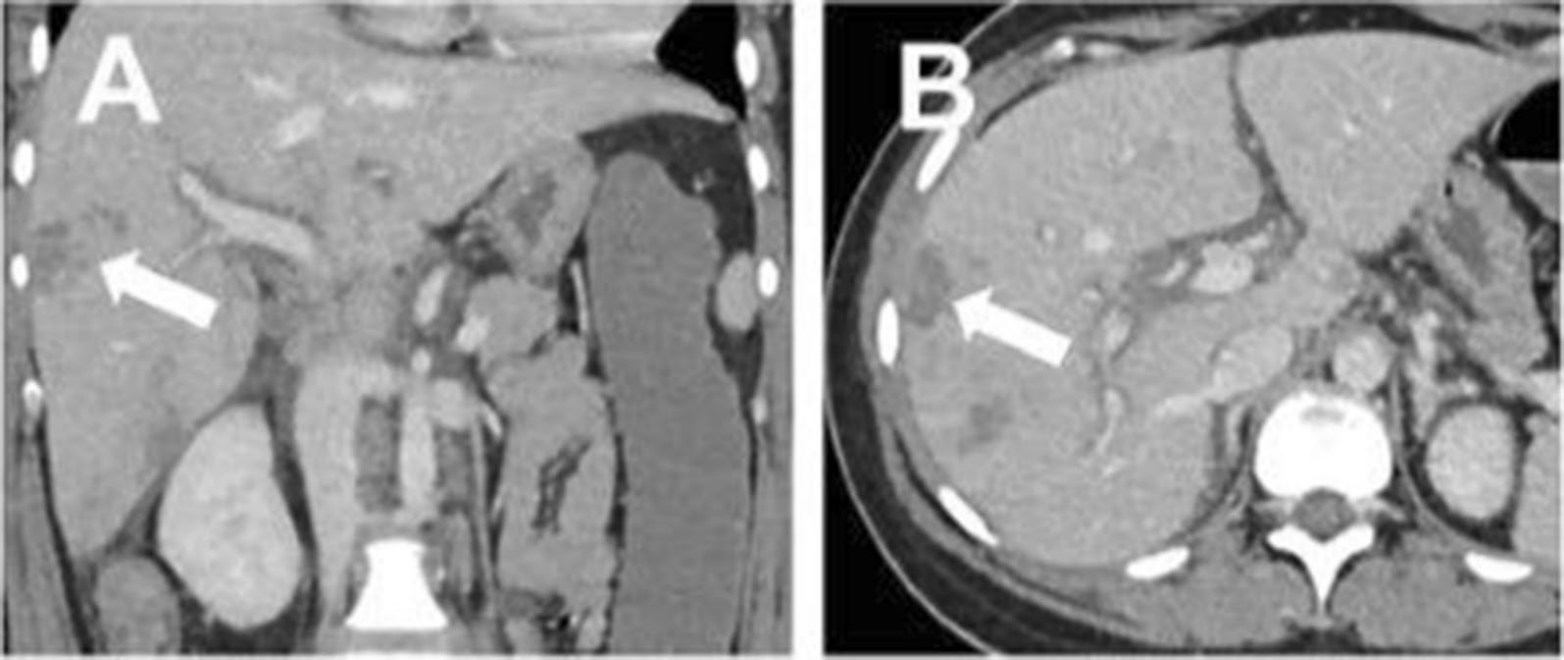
Representative images of an abdominal CT scan. Frontal (A) and transverse planes (B). There were multiple liver lesions, with the largest measuring 3 cm in diameter (arrows). *CT scan* = computed tomography scan
